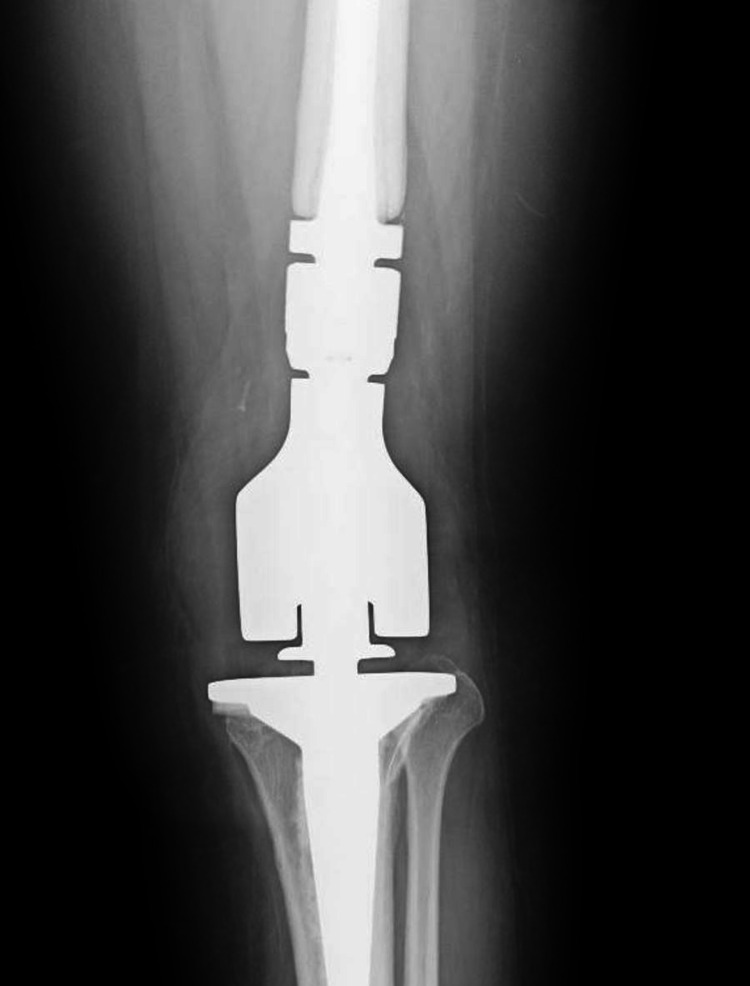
Anteroposterior radiograph of a 30-year-old woman (patient one) who underwent revision surgery, including wide excision and limb reconstruction after arthroscopic partial excision and debridement of a primary synovial sarcoma.
